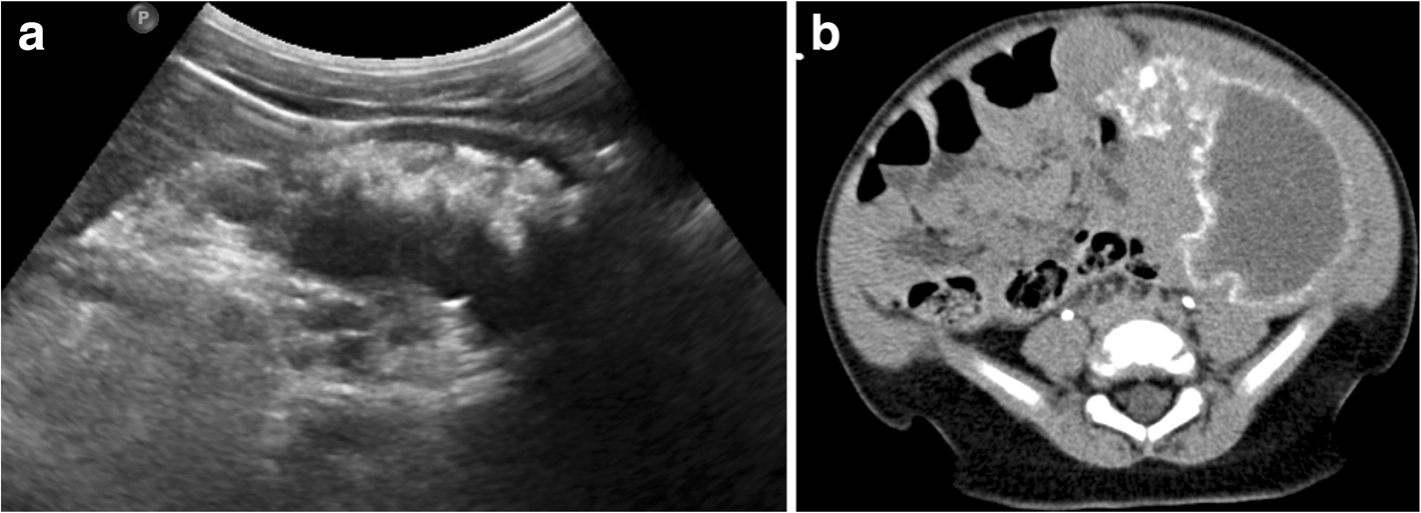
Splenic necrosis in pediatric Gaucher disease. Transverse grayscale abdominal ultrasound (a) of a 2-year-old male Gaucher disease patient with marked splenomegaly shows replacement of normal splenic parenchyma with fluid and hyperechoic regions corresponding to dystrophic calcification. Contrast-enhanced abdominal CT (b) of this patient demonstrates enlarged spleen replaced with liquefying necrosis and peripheral dystrophic calcifications
